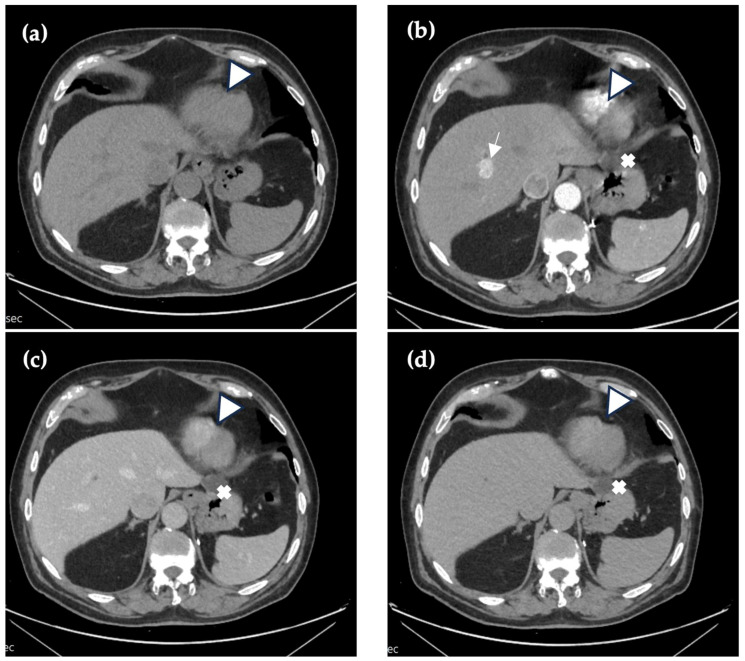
Forty-one months after first imaging, CE-CT revealed hyperdense lesions in the arterial phase (arrow) (b) with a slight increase in size, which can be characterized as a chylous component. In native (a), venous (c) and 5 min delayed (d) phases, the lesion demonstrates homogeneous hypoattenuation relative to the surrounding liver parenchyma. A hypoattenuating hepatic cyst in segment II (triangle) and a renal cyst (cross) can be visualized in native, arterial, venous, and 5 min delayed phases.
